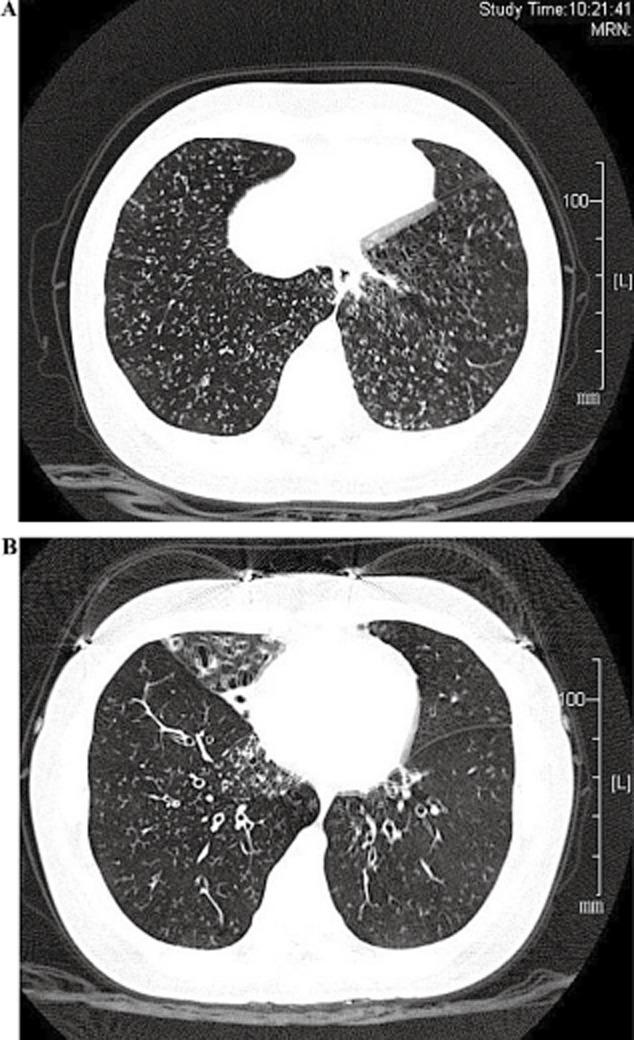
High-resolution computed tomography (HRCT) images after 20 days of azithromycin therapy. (A) Nodular shadows were obviously attenuated, (B) but bronchiectasis did not change.
